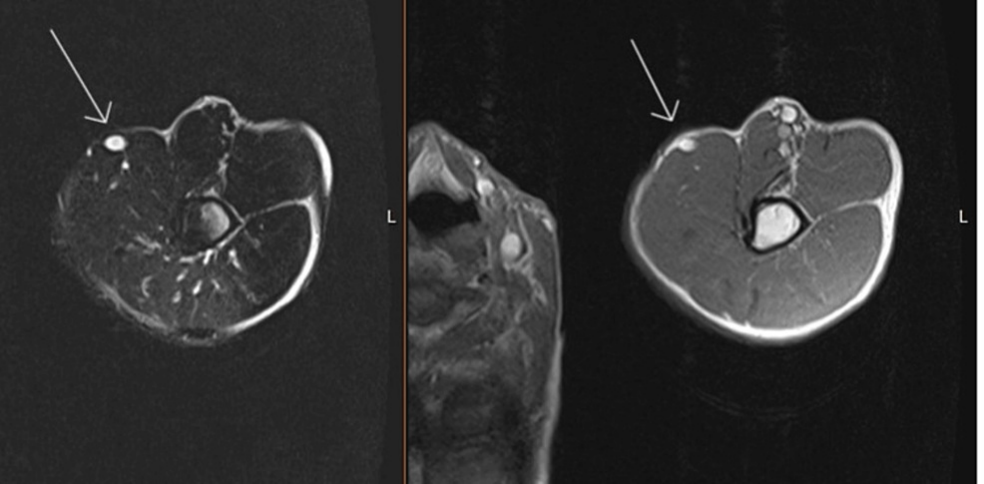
Soft tissue MRI of the chest The left side of the image shows a T2-weighted transverse section; the right side is the T1-weighted image taken in May 2021. A hyperintense lymph node is exhibited in both images in the 11 o'clock position.
